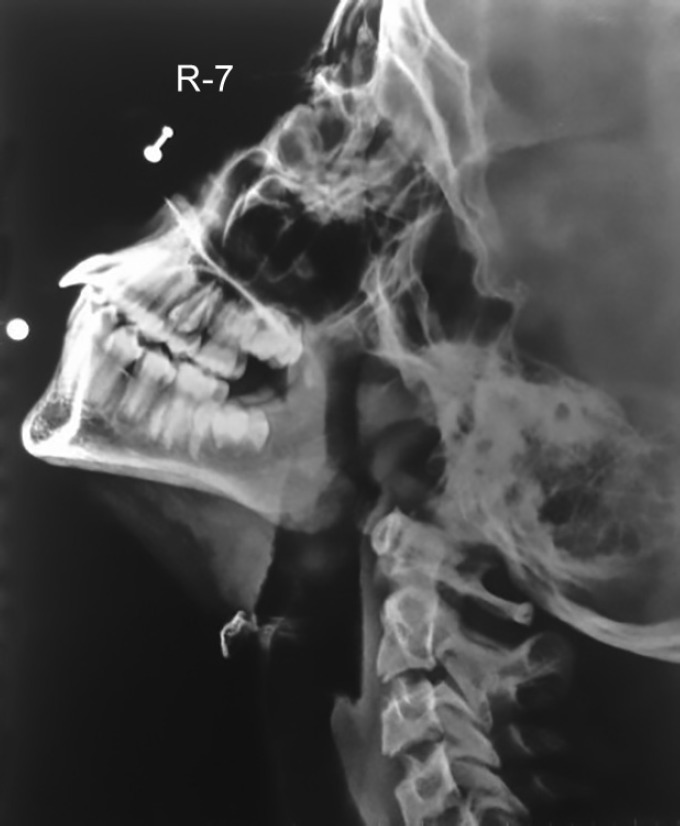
Lateral cephalometric radiograph of the nasopharynx showing enlargement of the adenoid gland which causes narrowing of the air passage
